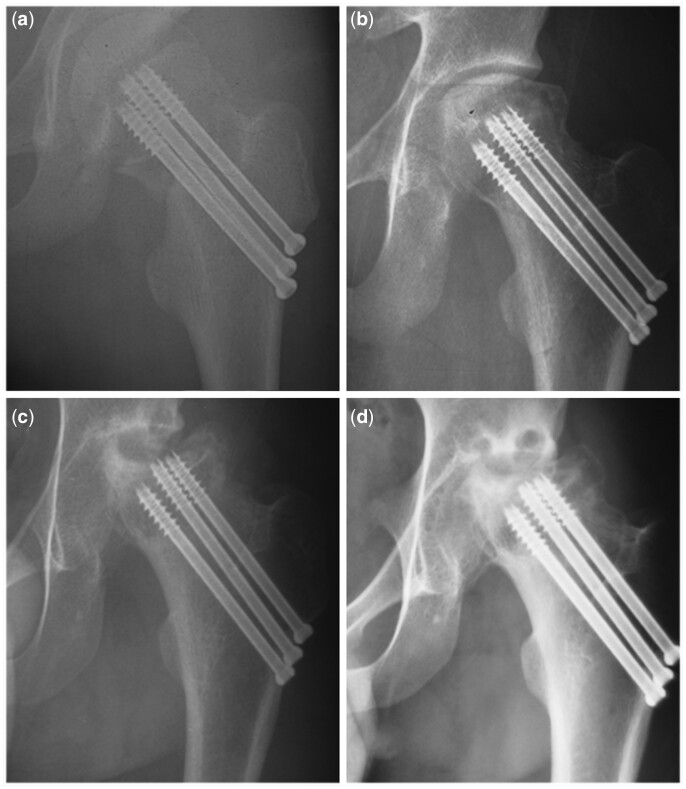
Adolescent of TFHN at collapsed stage showed an increasingly progression towards severe hip deformity and degeneration in the short term, if the subluxation emerged. A male patient of 16 years old, with left FNF, treated with three cannulated screws (a). TFHN (JIC classification C2) was diagnosed 1 year after FNF, which already progressed to collapsed stage with subluxation (b). During observational treatment, radiographs showed progressively femoral head collapse and subluxation 11 months after diagnosis of avascular necrosis (c). At latest follow-up, 2 years after FNF, left hip showed poor outcome according to Ratliff’s clinical criteria, with severe hip deformity (Stulberg classification IV) and hip degeneration (Tönnis grade III).
